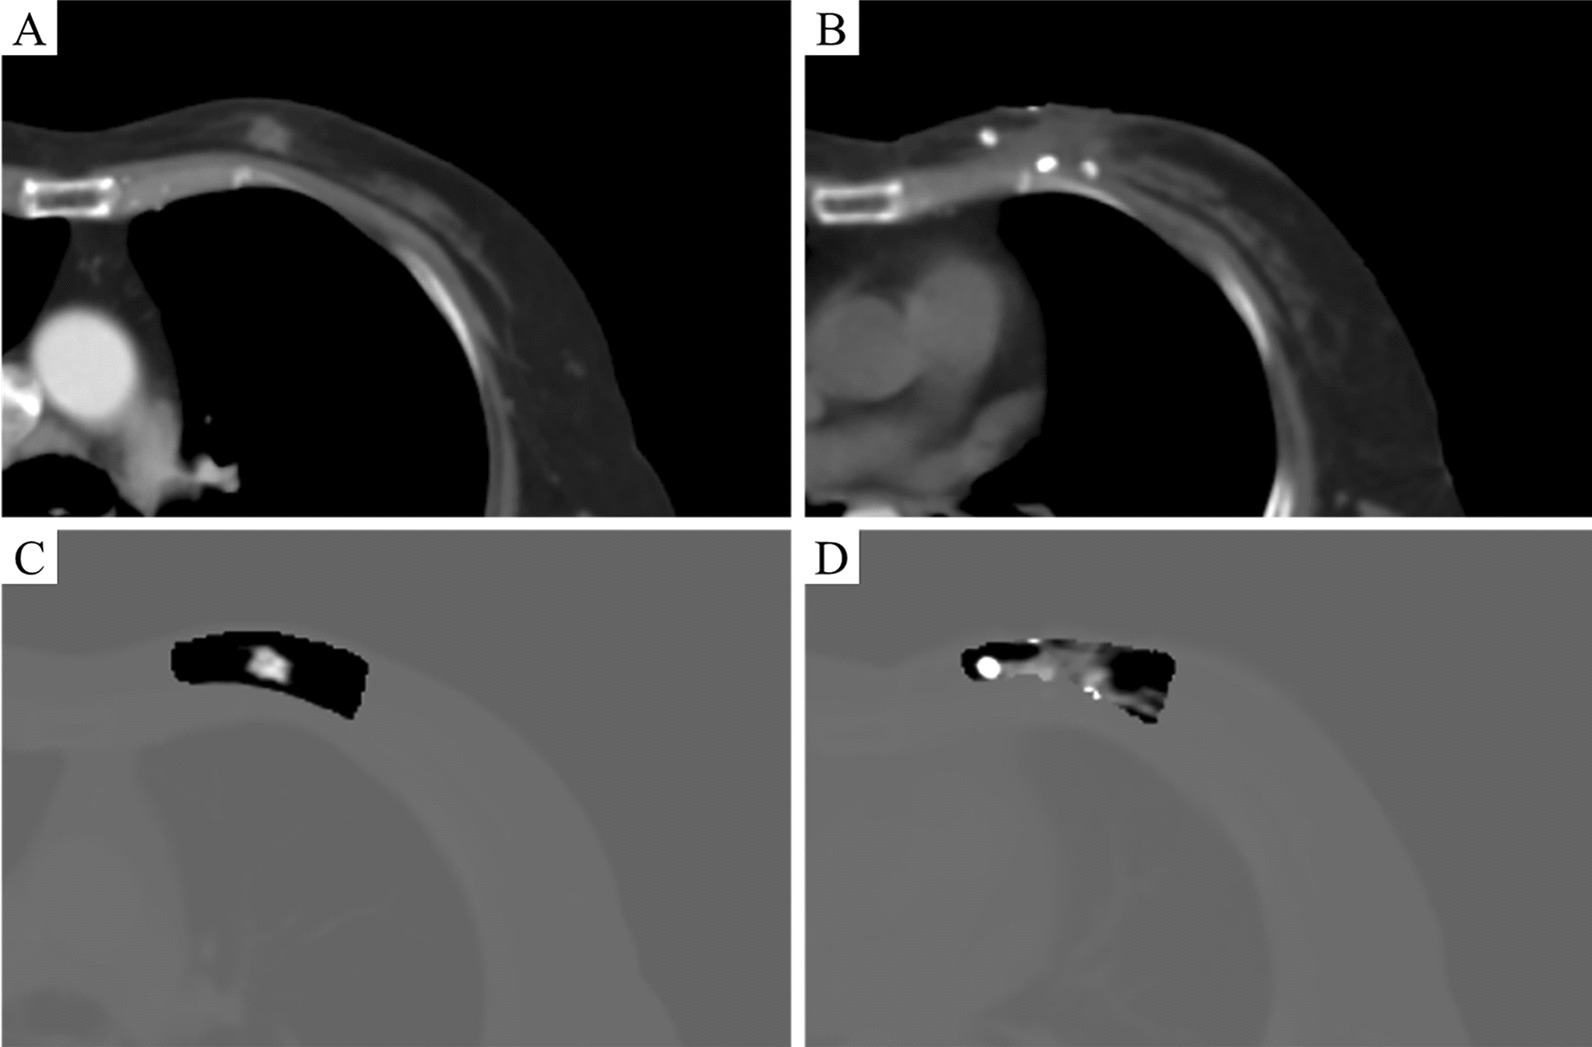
The effect of CT images before and after image enhancement. A The preoperative CT and B postoperative CT before image enhancement. C The preoperative CT and D postoperative CT after image enhancement
